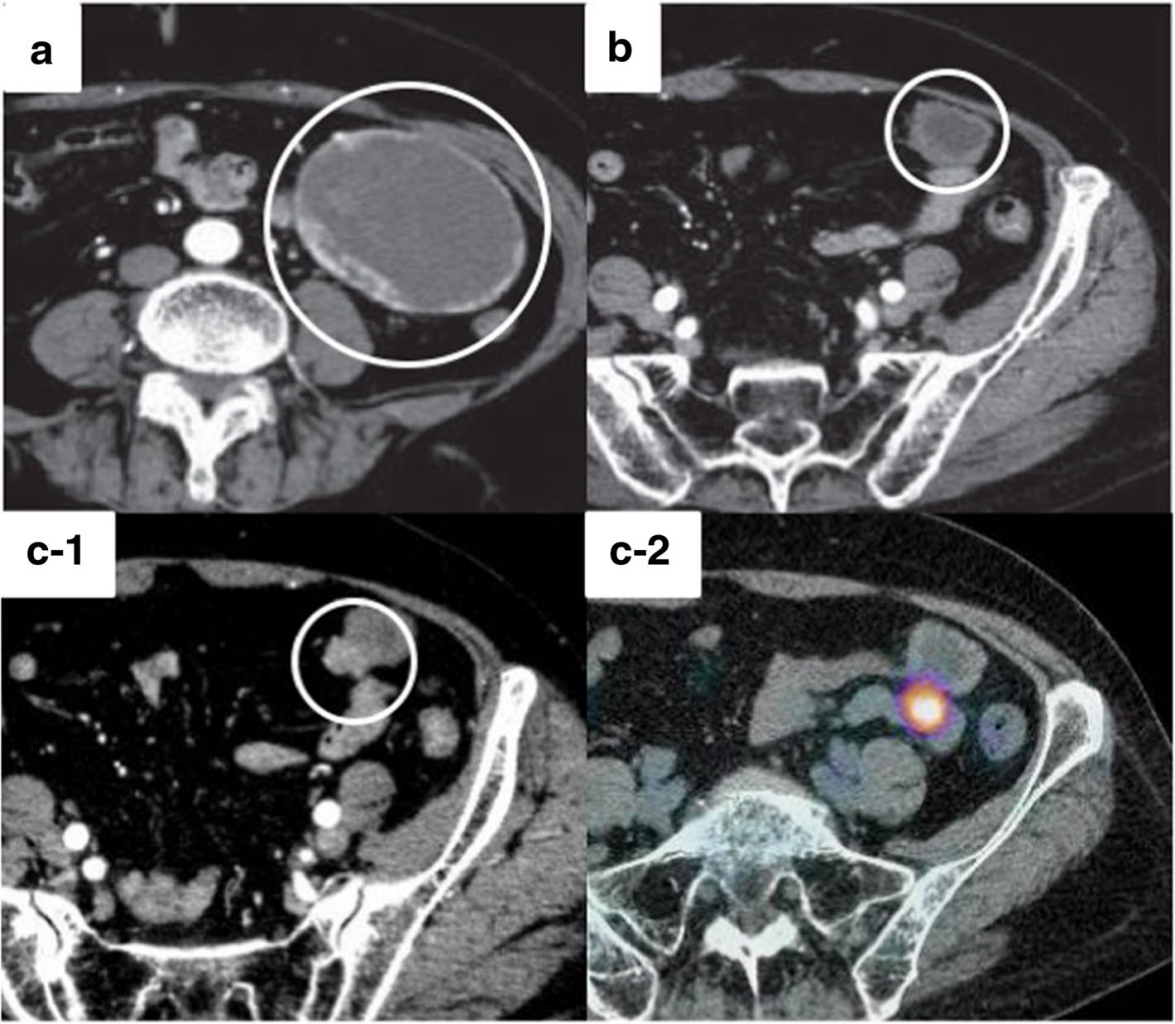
Case 2 imaging findings. a Abdominal CT at study enrollment. b Abdominal CT 3 months after start of nilotinib therapy. c Abdominal CT (C-1) and PET-CT (C-2) of the developing nilotinib-resistant tumor
